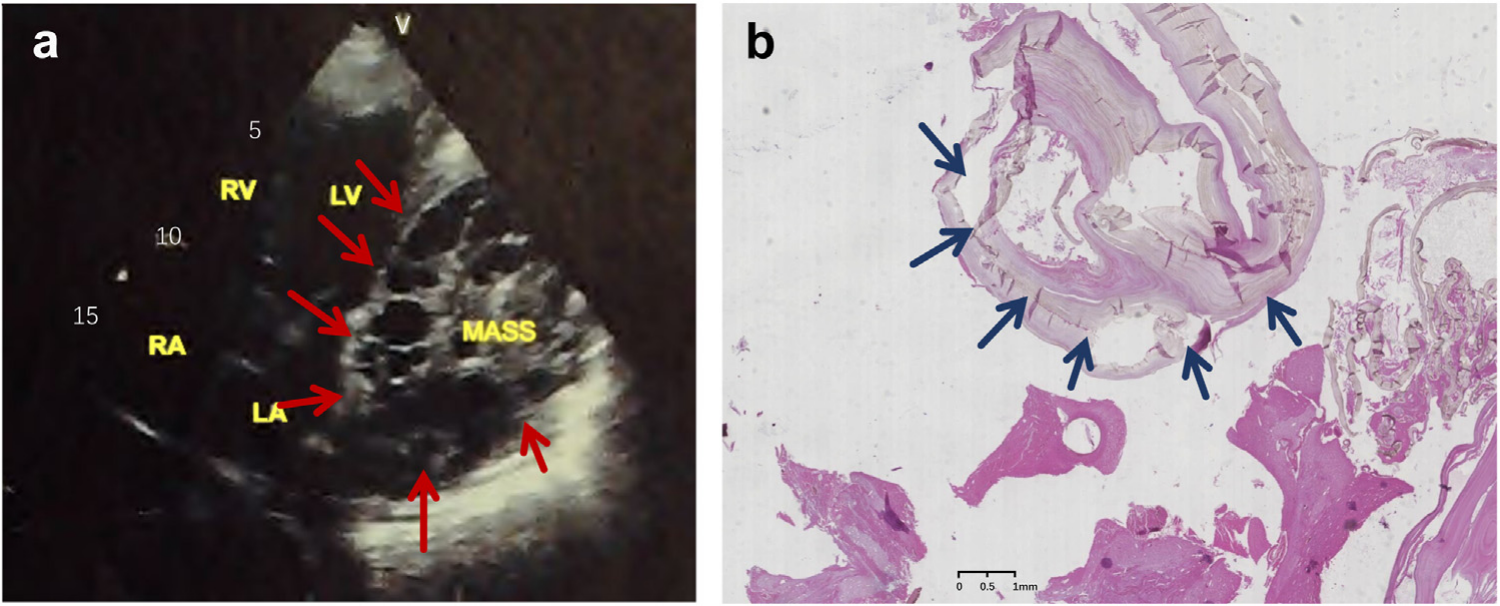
a: Preoperative echocardiogram; red arrow points to the hydatid cyst. b: Pathologic picture; blue arrow points to the endocyst. LA = left atrium; LV = left ventricle; RA = right atrium; RV = right ventricle.
